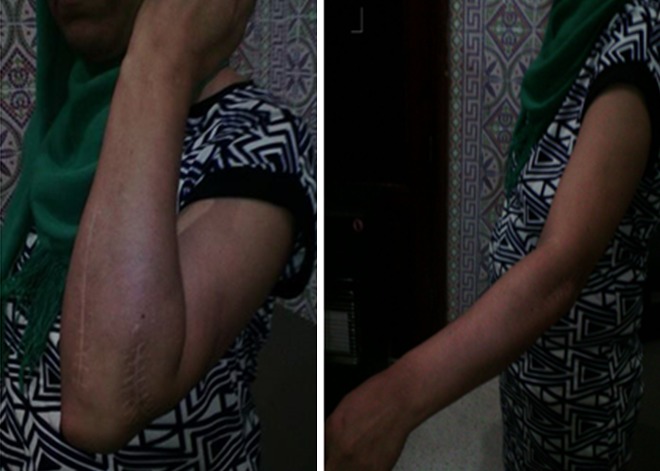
Radiographs obtained 2 years later showing a consolidated fracture of the radial styloid
